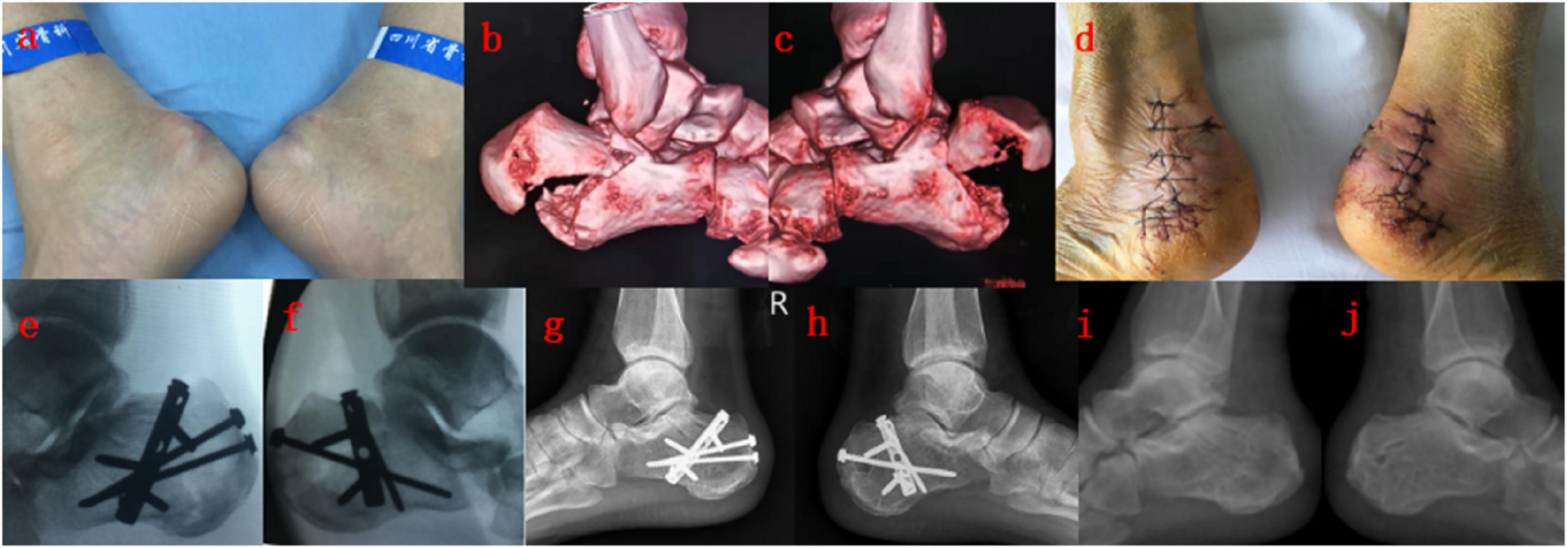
(a–c) A 45-year-old man suffered bilateral beak fracture when jumping from high altitude, he underwent emergency surgery, to whom open reduction and internal fixation with “L-shape” plate were conducted; (d) incision condition at discharge; (e,f) intraoperative radiograph; (g,h) x-ray radiograph at 11 months after surgery; (i,j) after removing the internal fixation.
